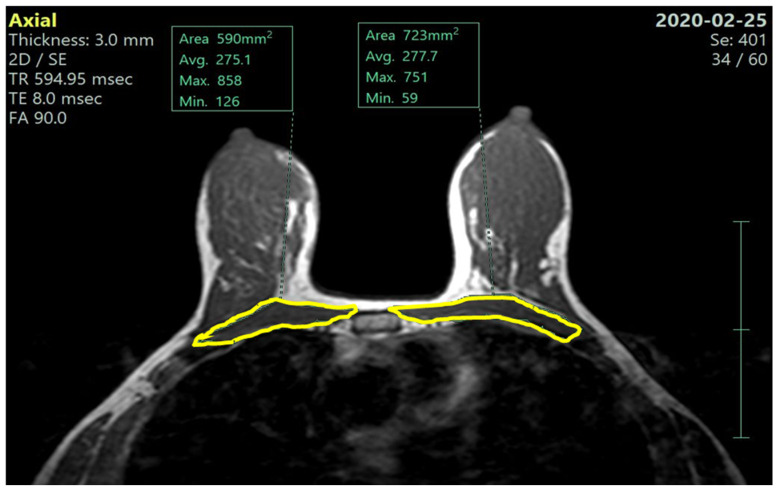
Post-NACT left and right pectoralis muscle areas (MRI—axial T1 non-fat sat image).
